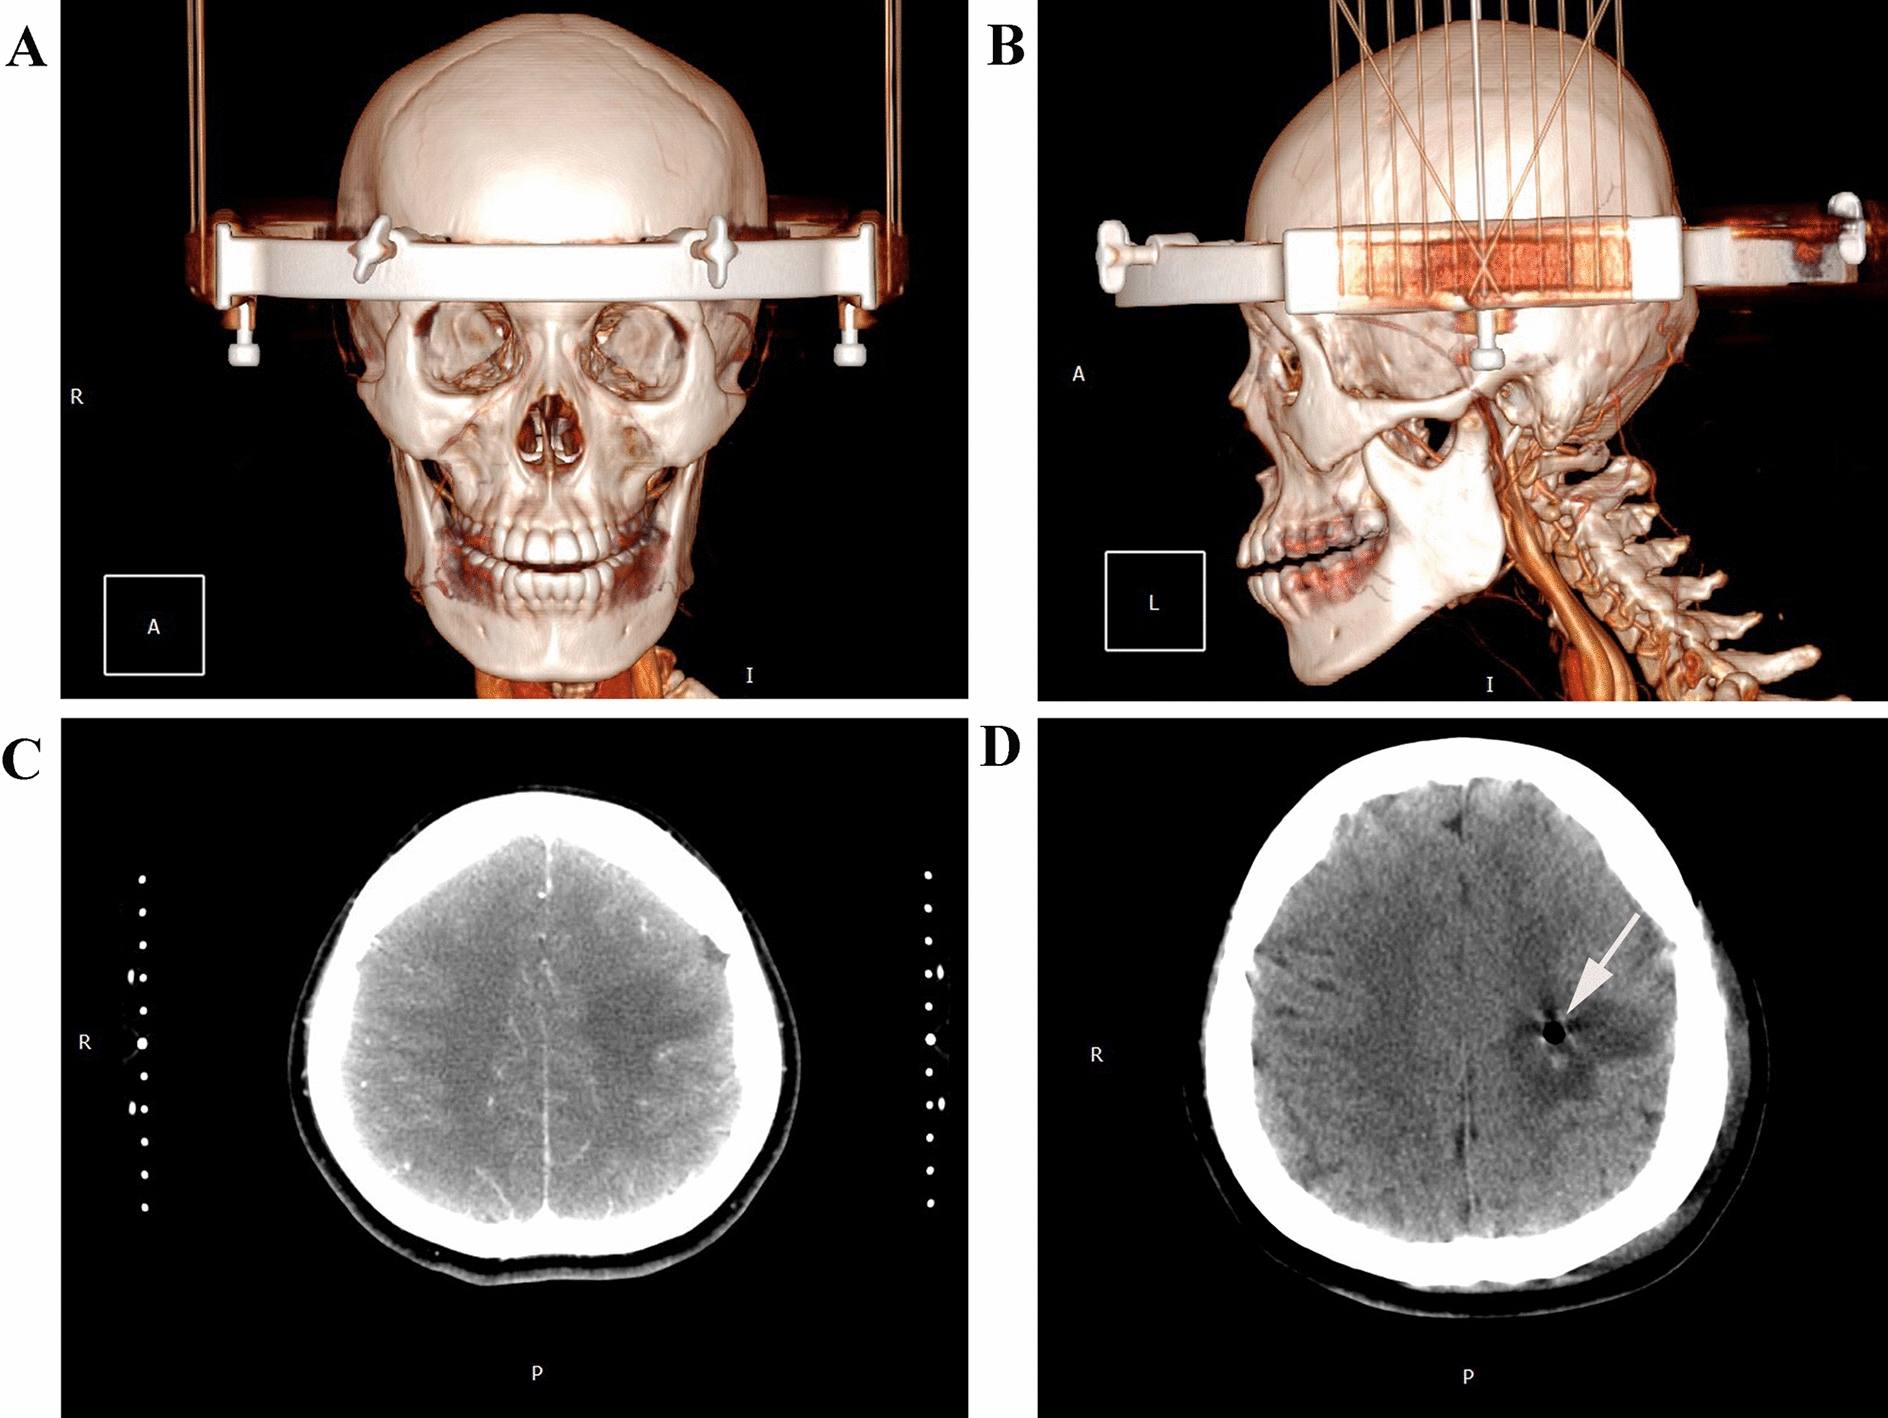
Three-dimensional (3D) computed tomography (CT) images before and after stereotactic biopsy surgery. A Localization of the area for stereotactic surgery and an axial image of the 3D CT reconstruction. B Stereotactic biopsy localization and sagittal image of the 3D CT reconstruction. C Stereotactic biopsy targeting the left frontal lesion. D CT scan of the head, 1 day after stereotactic biopsy, showed a shadow indicating gas present in the left frontal lesion (white arrow)
